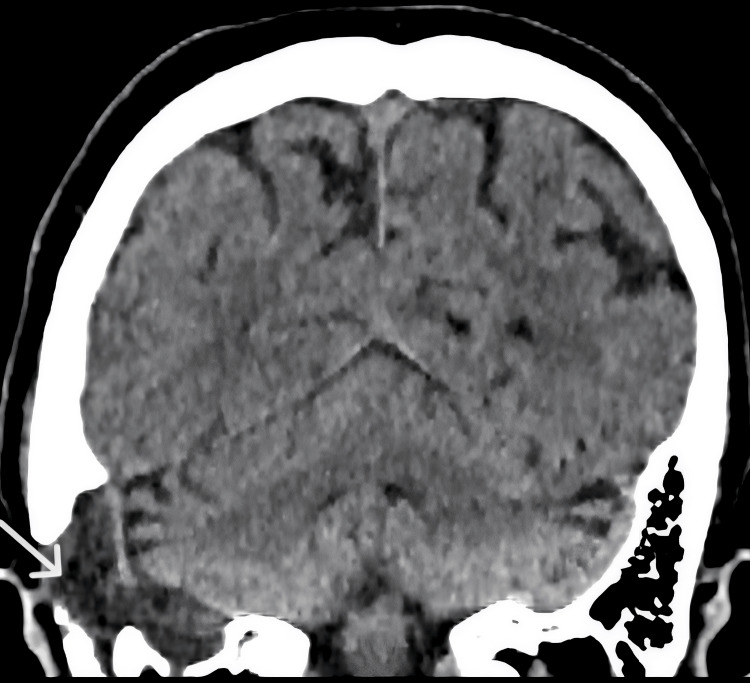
Unenhanced coronal CT image demonstrating a low-density lesion involving the right mastoid (white arrow) with associated bony erosion. The lesion is seen in proximity to the mastoid segment of the facial nerve canal
